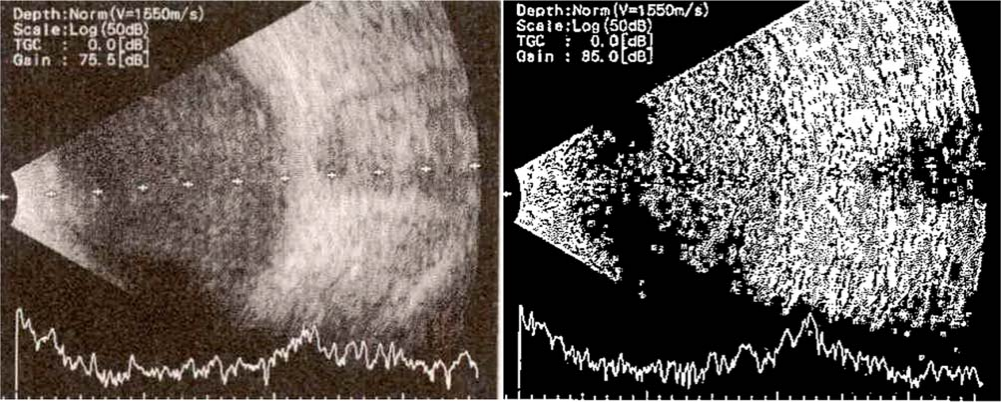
B-scan ultrasound demonstrating moderate vitreous echoes and vitreous abscess in patient 4.
